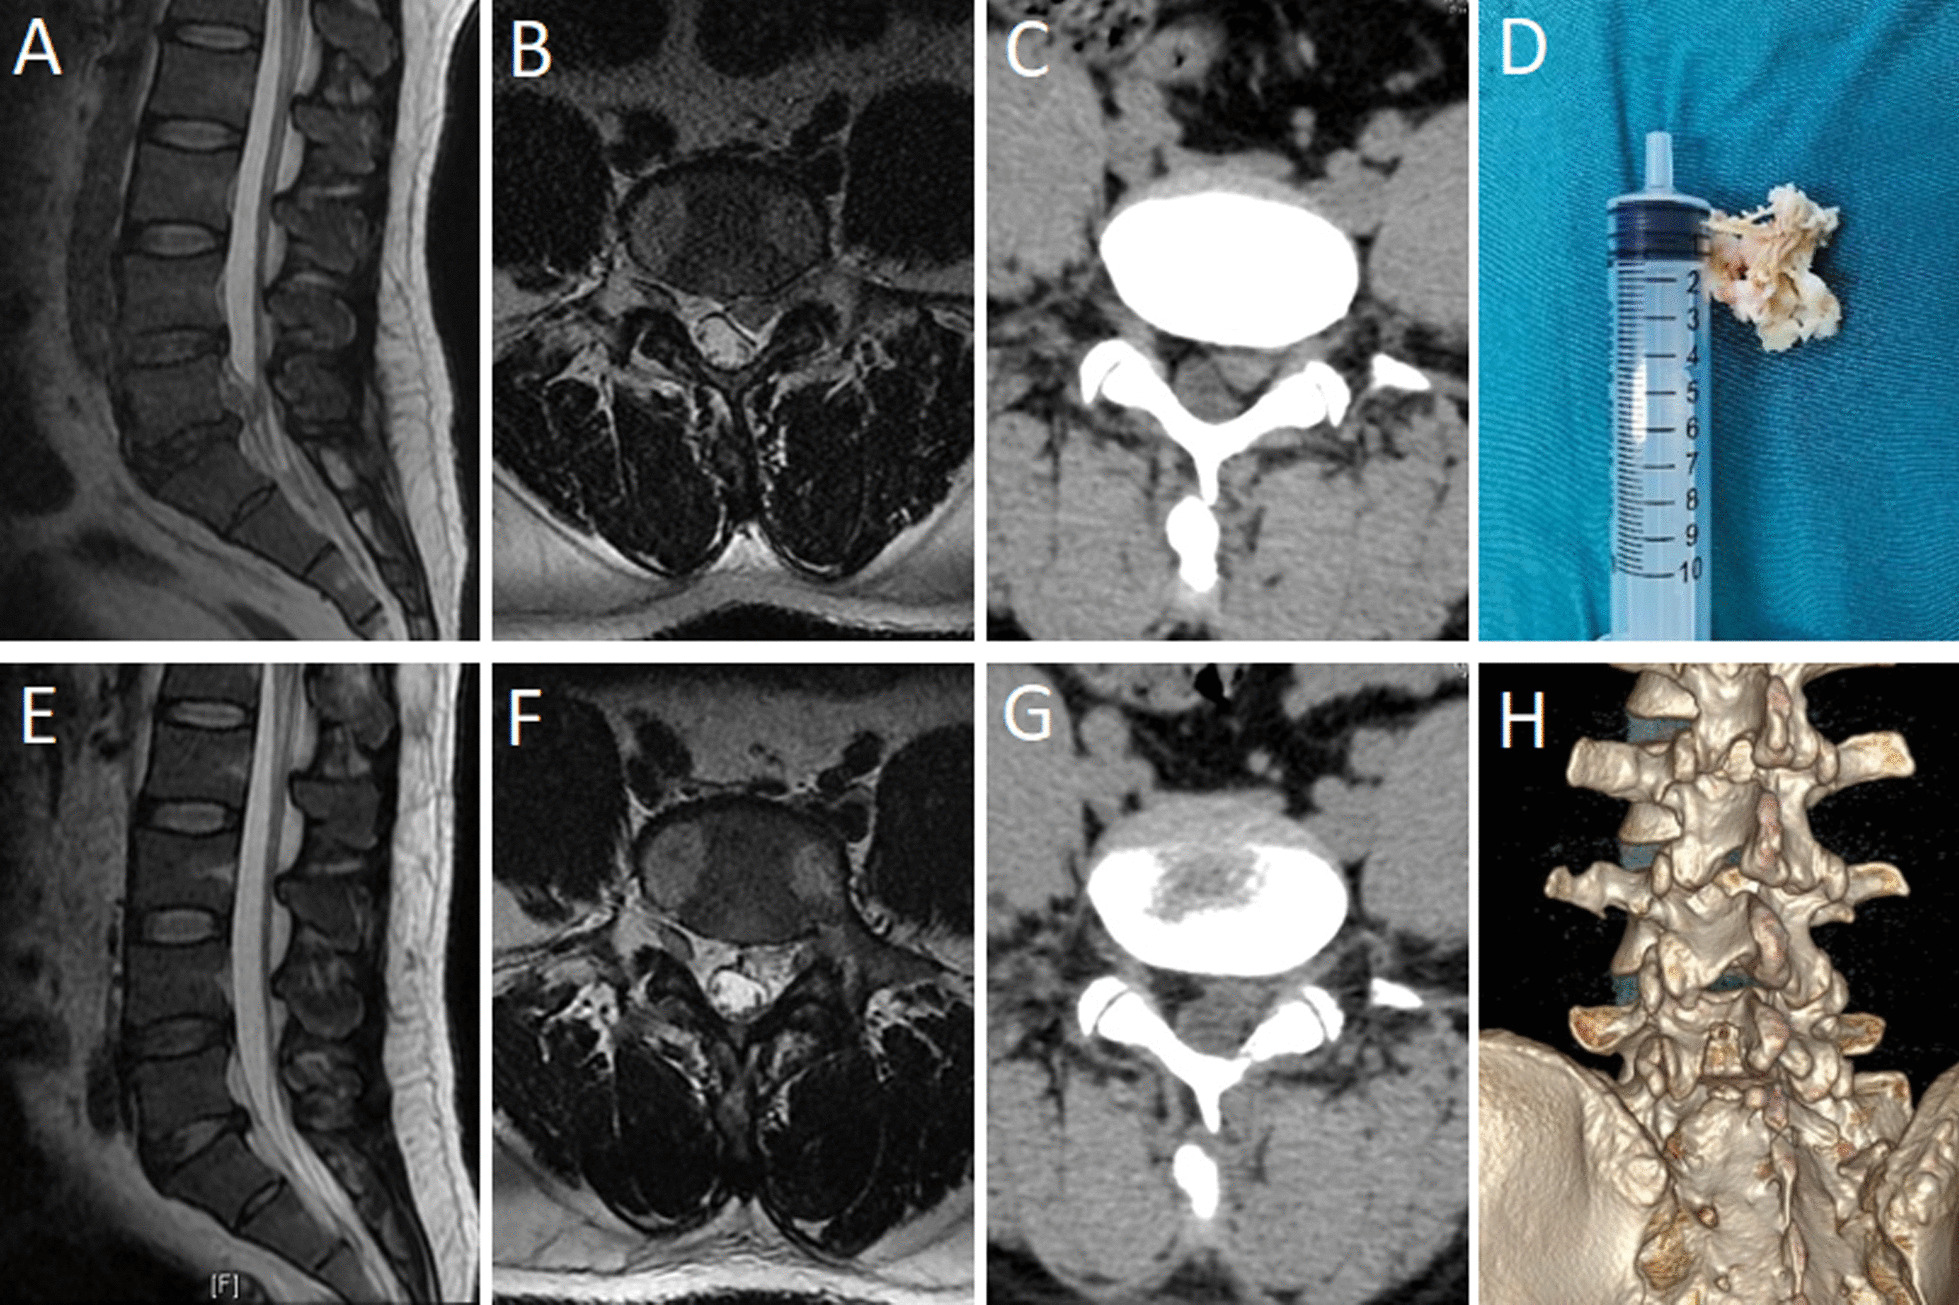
Patient, male, 53 years old, L5-S1 lumbar disk herniation, treated with UBE technology. A–C Preoperative MRI and CT showed L5-S1 intervertebral disk herniation, upward migration of the nucleus pulposus, and compression of the dura and nerve roots. D Removed nucleus pulposus tissue. E, F Postoperative MRI showed that migrated nucleus pulposus tissue was removed. G, H Postoperative 3D CT showed preserved facet on the surgical side and limited bone resection
